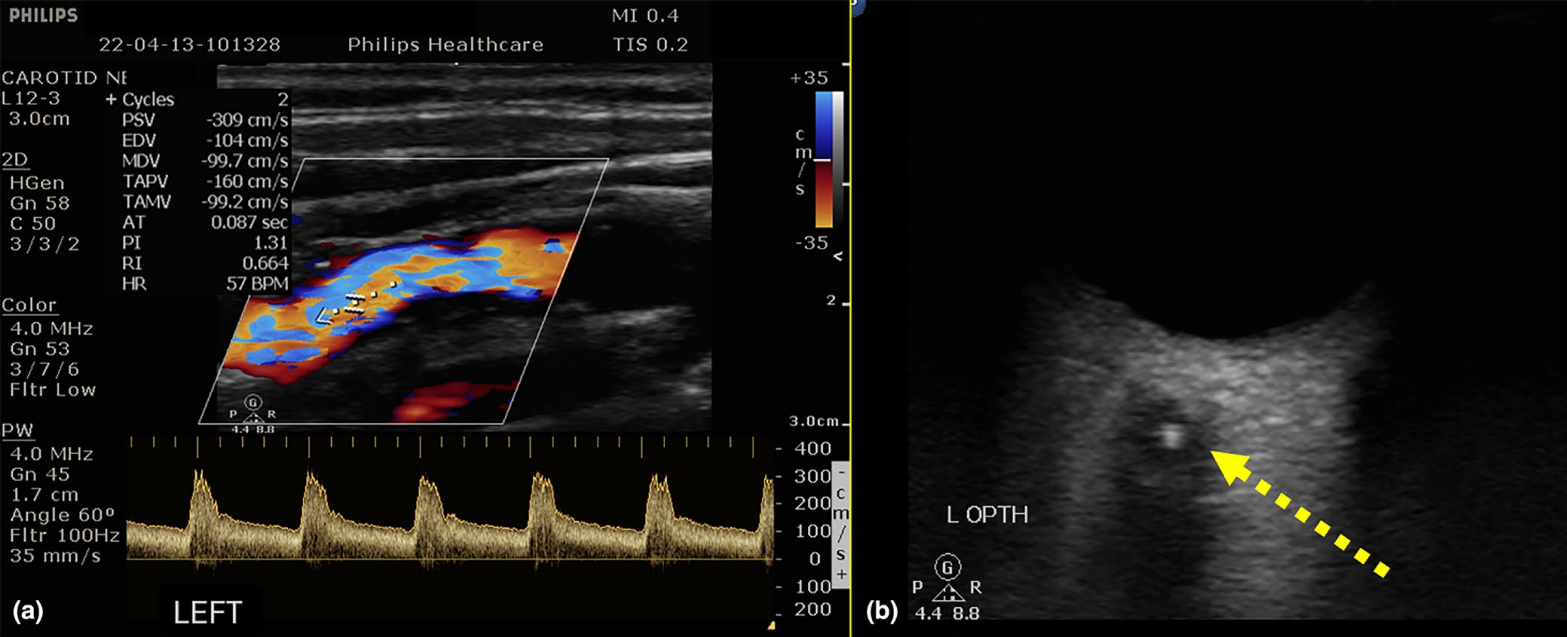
Patient with amaurosis fugax due to symptomatic internal carotid artery stenosis, showing a hypoechoic plaque with a peak systolic velocity of 309 cm/sec causing 70%–90% stenosis of the left internal carotid artery (a) with hyperechogenic retrobulbar spot sign in the central retinal artery as detected by Transorbital Sonography (b).
